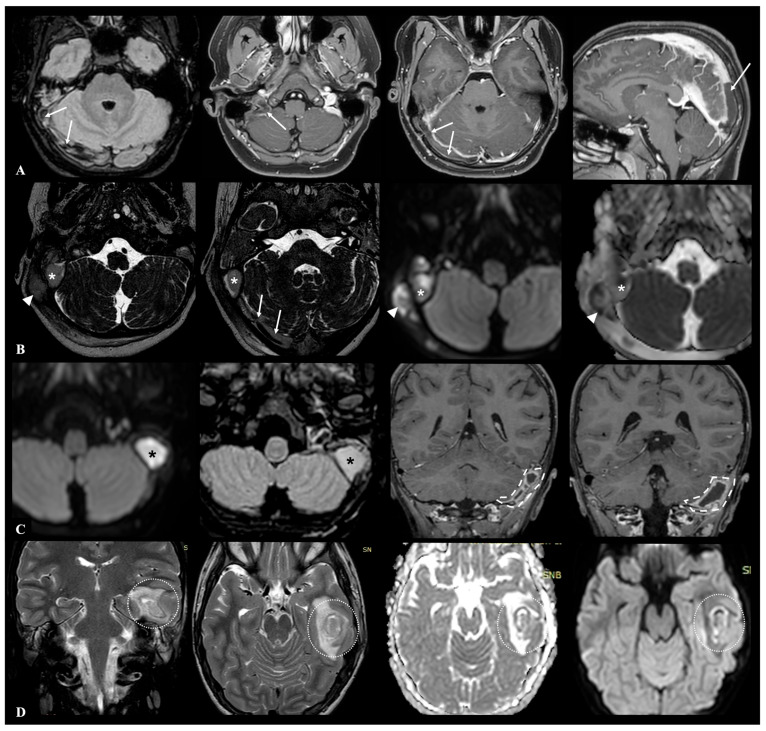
MRI examples of the major possible otogenic intracranial complications in pediatric AOM patients: row (A) thrombosis of the transverse-sigmoid-jugular venous complex extending to superior sagittal sinus (white arrows) in a 13-year-old male patient with right-sided ACOM; row (B) right-sided ACOM in a 7-year-old female patient, with both extracranial extension (retromastoid abscess located beneath the proximal insertion of the sternocleidomastoid muscle—white arrowheads) and intracranial extension (epidural empyema of the posterior cranial fossa—white asterisks—associated with severe flow reduction in the transverse sinus—white arrows—and meningeal thickening); row (C) left-sided posterior cranial fossa subdural empyema (white dashed lines) in a 5-year-old male patient with large otomastoid abscess (black asterisks) and meningeal thickening; row (D) left middle cranial fossa brain abscess (white dotted circles) involving the temporal lobe secondary to ACOM in a 4-year-old female patient.
